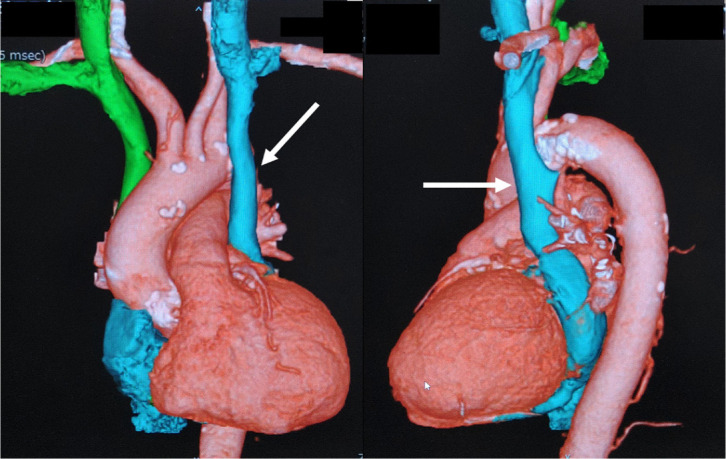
Three-dimensional computed tomography angiography. Arrows indicate
